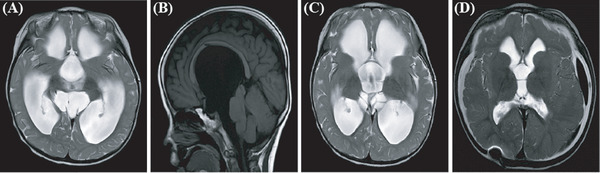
Images of patient 2. (A) Magnetic resonance imaging (MRI)‐T2WI sagittal image and (B) coronal image showing hydrocephalus prior to ventriculoperitoneal shunt surgery; (C) Post‐shunt MRI image showing enlargement of the suprasellar cistern arachnoid cyst; (D) Following ventriculostomy, the suprasellar cistern cyst disappeared, but right subdural effusion and left subdural hematoma were observed.
